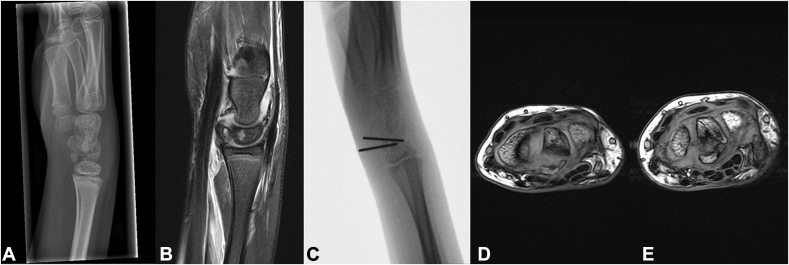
A Wrist radiograph one day after injury of the right wrist, showing a displaced lunate fracture. B T2-weighted MRI of the right wrist one day after injury, revealing the lunate fracture but no additional ligament injury. C Intraoperative lateral view after placing two K-wires to fix the fracture fragments. D Approximately 16 months after injury, T1-weighted MRI shows no enhancement of the lunate, possibly indicating inadequate blood perfusion. E At 28 months after injury, T1-weighted MRI reveals no consolidation yet of the lunate fragments.
